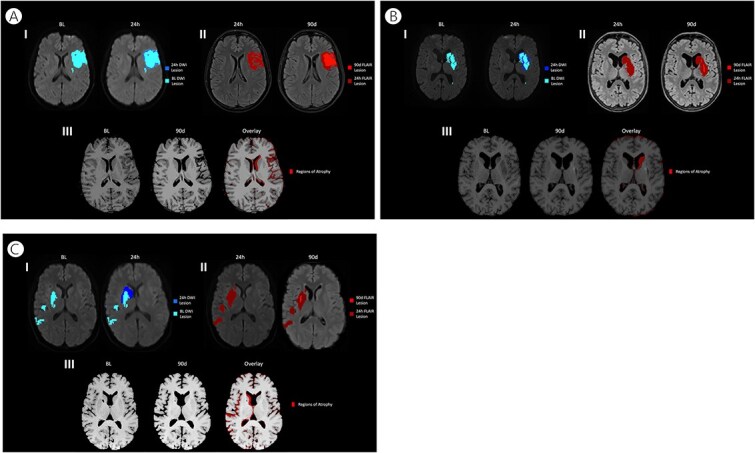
Examples A–C demonstrating the relationship of the acute DWI lesion, 24 hours FLAIR stroke, and the regional brain atrophy over 90 days. For each example, the colour legend indicates I) DWI at baseline (teal) and growth at 24-hours post-stroke (dark blue). II) FLAIR images at 24-hour (red) and 90-days post-stroke (dark red). III) T1-weighted imaging at baseline and 90-days post-stroke, with the regions of brain volume loss shown in red. 2A) Example imaging of a 49-year-old female with left-sided M1 stroke with a baseline NIHSS of 11. She underwent endovascular therapy (EVT) without tPA with a resultant eTICI of 2C and received NA1. 2B) Example imaging of a 66-year-old female with a left-sided M1 stroke with a baseline NIHSS of 12. She underwent EVT with tPA with a resultant eTICI of 3 and received NA1. 2C) Example imaging of a 44-year-old female with a right-sided M1 stroke with a baseline NIHSS of 16. She underwent EVT without tPA with a resultant eTICI of 2B and received placebo.
